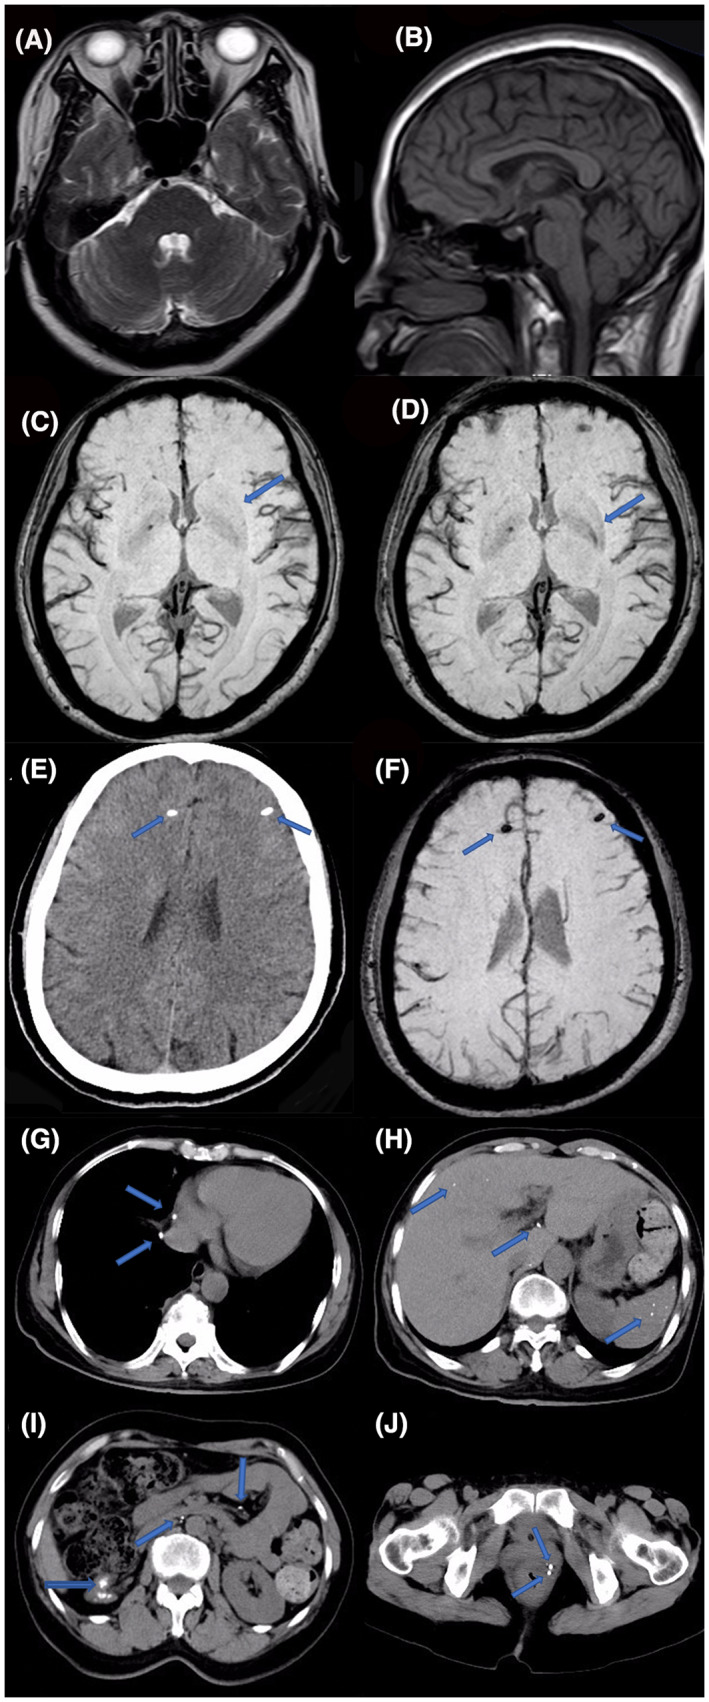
Brain computed tomography (CT) and magnetic resonance imaging (MRI) findings; brain susceptibility weighted imaging (SWI) sequence of putamen; chest, abdominal, and pelvic CT findings. Axial and sagittal brain MR of the patient showed no abnormality. Axial brain MRI showed no “Hot cross bun” (A) and sagittal brain MRI showed no infratentorial atrophy (B); no obvious putaminal signal changes were observed before the onset of symptom of MSA‐P in 2020 (C, blue arrow). The left putaminal hypointensity observed in 2021 (D, blue arrow). Multiple calcifications in frontal lobe on cerebral CT and SWI sequence, Multiple calcifications (blue arrows) in frontal lobe on cerebral CT (E), and the multiple calcifications (blue arrows) in the same place on SWI sequence (F). Multiple calcifications (blue arrows) in inferior vena (G), liver, spleen (H), abdominal cavity, right kidney (I), and bladder (J).
